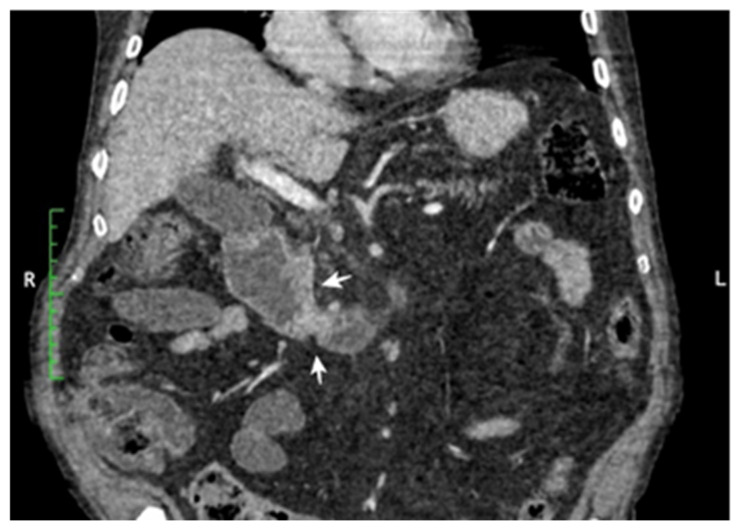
Coronal CT reconstruction post-contrast: infiltrative tumor located on the upper part of the small bowel, with circumferential distribution. Small lymph nodes are visible. Pathology revealed duodenal adenocarcinoma.
